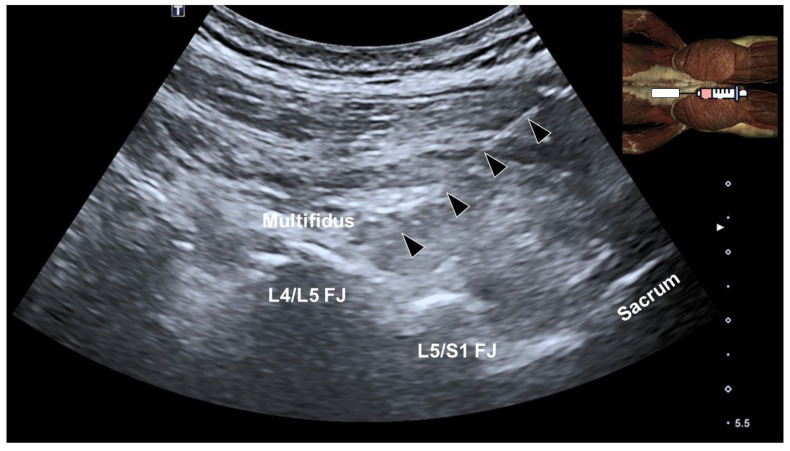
Ultrasound-guided injection into the lumbar facet joints and the overlying multifidus muscle in the sagittal plane. Arrowheads, needle trajectory; FJ, facet joint.
